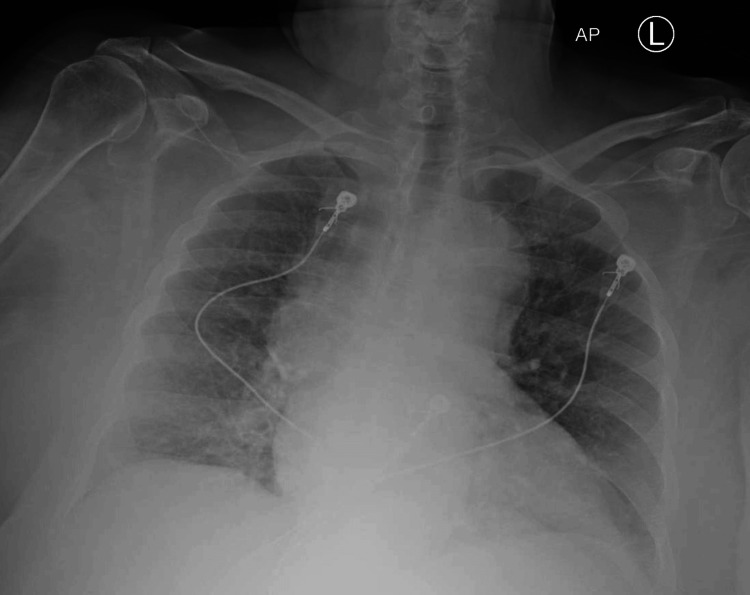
Anteroposterior chest radiograph demonstrating apparent mediastinal widening. Initial chest radiography demonstrated apparent mediastinal widening on portable anteroposterior imaging, prompting concern for possible acute aortic pathology and subsequent computed tomography angiography evaluation.
